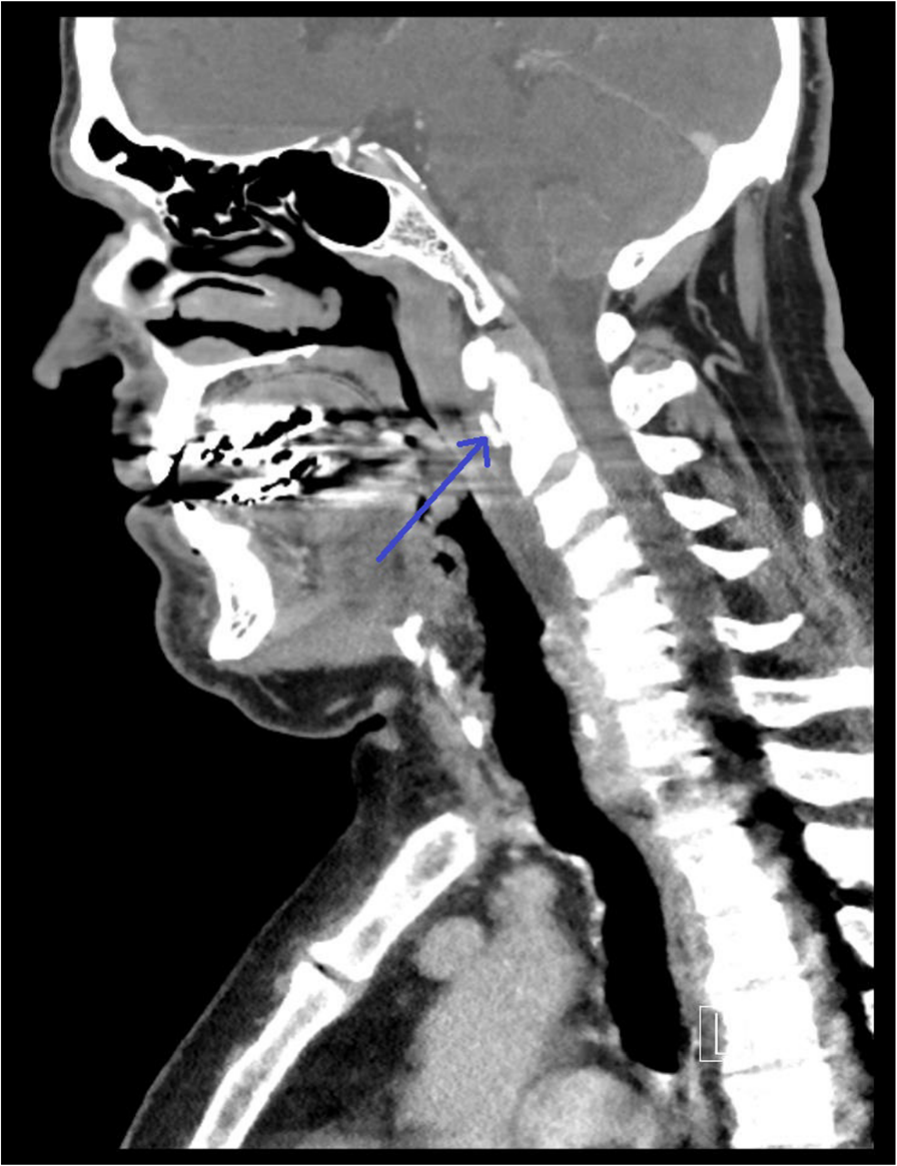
CT of soft tissues of the neck shows a hyperdensity from calcium deposition in the longus colli muscle at the level of C2 (blue arrow)
